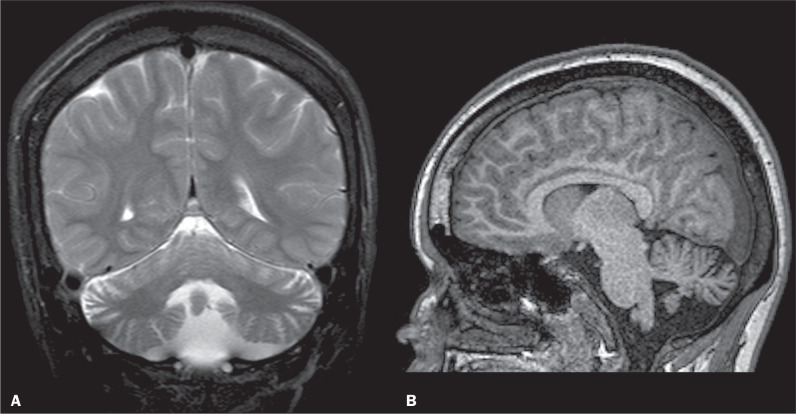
Coronal T2-weighted image (A) showing a reduction in the volume of the cerebellar parenchyma, with evident cerebrospinal fluid among the cerebellar folia, and enlarged fourth ventricle. T1-weighted image (B) showing calvarial thickening. The patient had a history of chronic phenytoin use.
